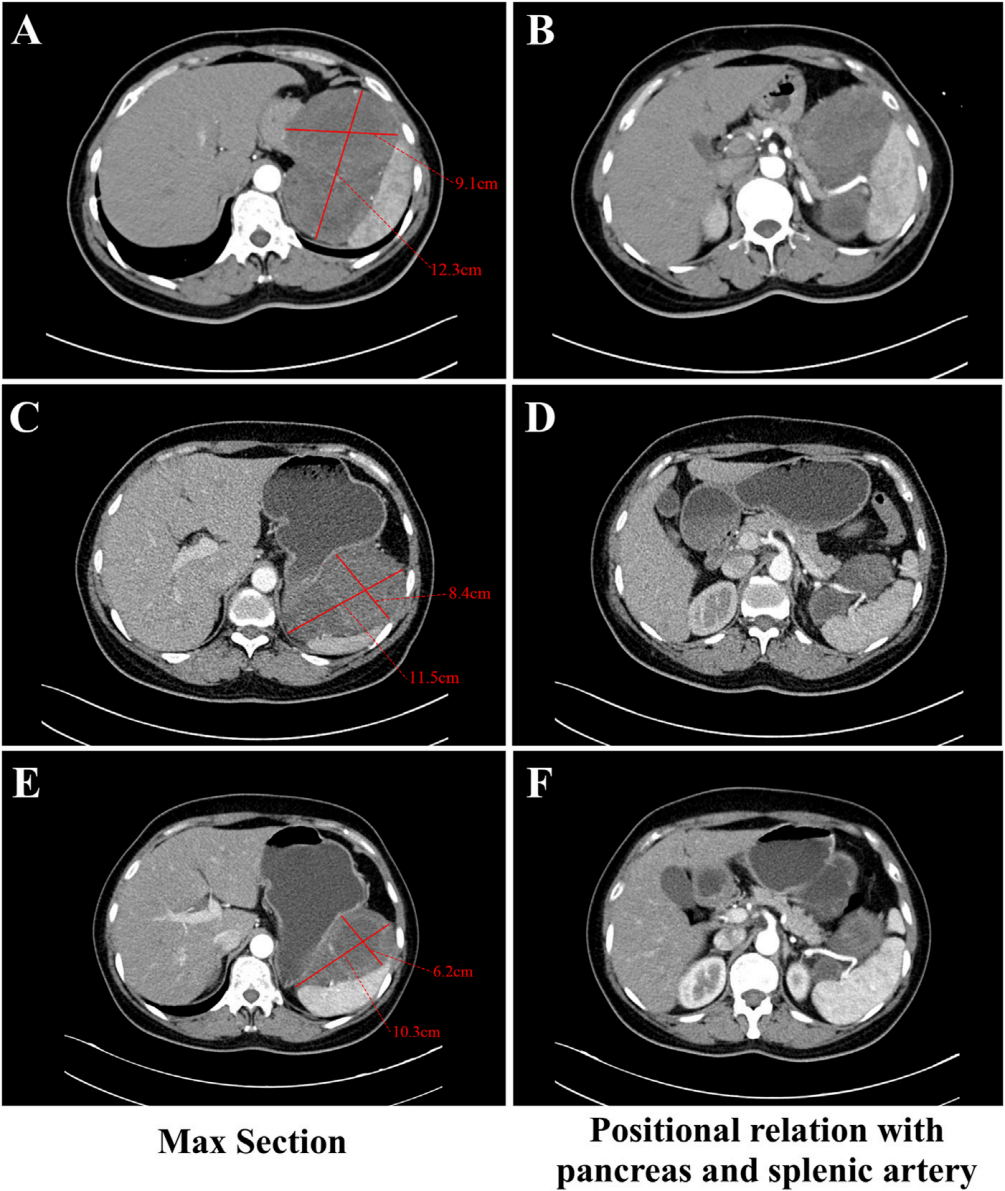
Abdominal contrast-enhanced CT during the arterial phase images with a thick-slice protocol (5 mm section thickness) for the GIST at different stages of therapy. (A, C, E) The section with the largest extent of the GIST in CT. Figure A showed the mass measuring 12.3 cm × 9.1 cm before any therapy. Figure C showed the mass measuring 11.5 cm × 8.4 cm at final follow-up with imatinib. Figure E showed the mass measuring 10.3 cm × 6.2 cm after taking Ripretinib for 5 months. (B, D, F) The relationship between the GIST and the pancreas and splenic artery in CT. (B) showed the mass was poorly demarcated from the pancreas locally and wrapped around the splenic artery before any therapy. (D) showed the mass was associated with the adjacent splenic artery and pancreas closely at final follow-up with imatinib. (F) showed clear partition from the pancreas and splenic artery after taking Ripretinib for 5 months.
